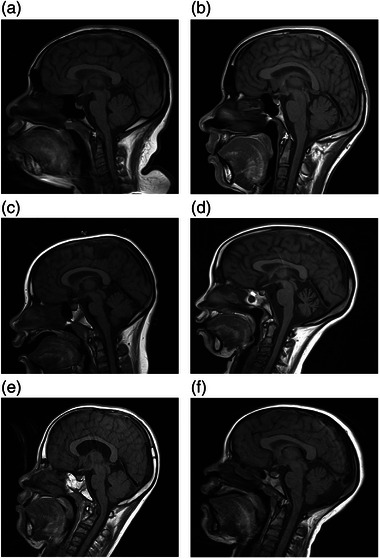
Sagittal T1/SE magnetic resonance imaging (MRI) view of (a) a 25‐year‐old adult male with Down syndrome (DS), (b) a 25‐year‐old male control participant, (c) a 12‐year‐old female with DS, (d) a 12‐year‐old female control participant, (e) a 14‐year‐old male with DS, and (f) a 14‐year‐old female with DS for comparison between DS and control participants as well as between DS males and females.
